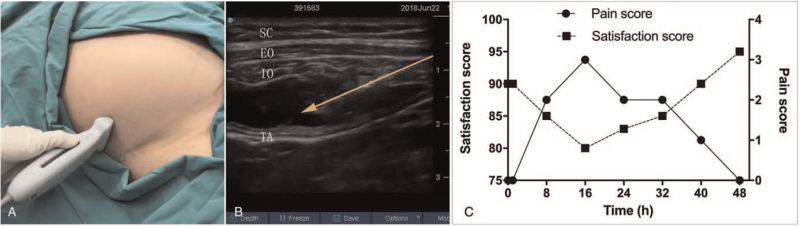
(A and B) Approach of ultrasound-guided lateral transversus abdominis plane (TAP) blocks. Orange arrow line indicates *the needle trajectory*. EO = external oblique, IO = internal oblique, SC = subcutaneous tissue, TA = transversus abdominis, TAP = transverse abdominis plane. (C) The outcome of pain and satisfaction score in actual patient data. The satisfaction score was evaluated according to the Leiden Perioperative Care Patient Satisfaction Questionnaire. Visual Analogue Scale (VAS) is recommended for the assessment of pain scores.
